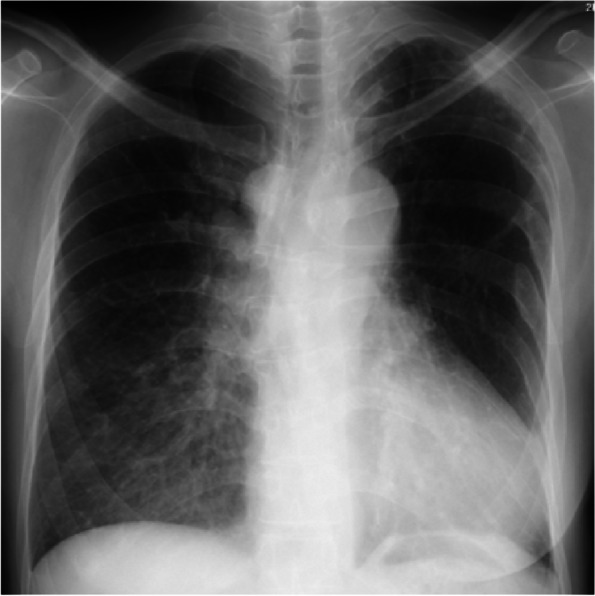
The anteroposterior chest radiography showed a boot-shaped heart and no pulmonary congestion
